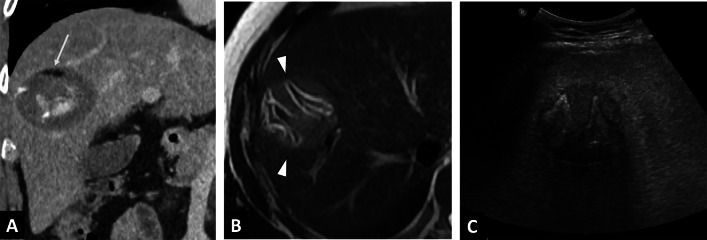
Cystic echinococcosis lesion CE4 with negative result on serology test. A CT image in the coronal plane obtained during the portal phase of enhancement: CE lesion of the right lobe with calcified membrane, leads to complementary exploration in MRI and US to assess activity. Note the macroscopic fat content (arrow). B T2-weighted MR image in the axial plane showing low signal in T2 and absence of daughter cysts (arrowheads). C B-mode ultrasound: echogenic structure of the CE lesion, without daughter cyst. Lesion classified as CE4
